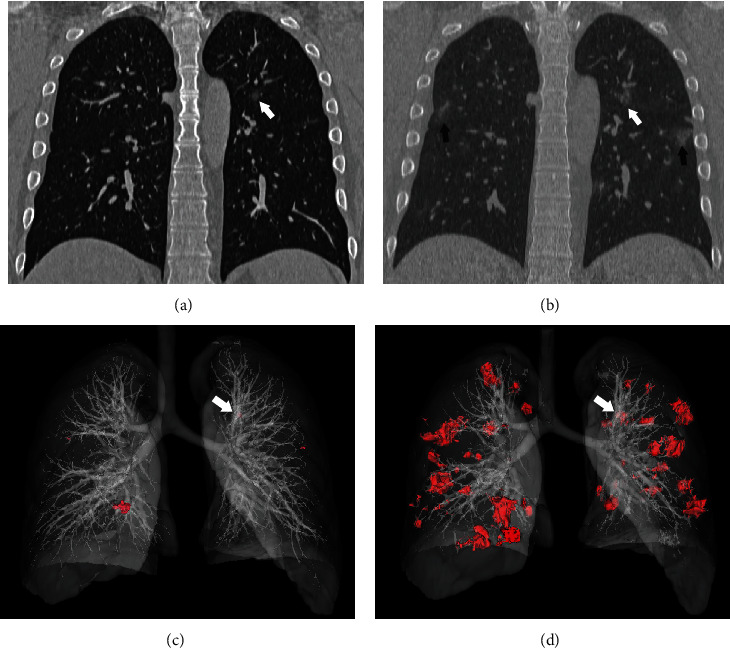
Imaging in a 25-year-old man who presented with fever and cough for 2 days. The coronal CT image shows a small ground-glass nodule (white arrow) in the apicoposterior segment of the left upper lobe (a). Three days later, the second CT image shows multiple nodular and patchy ground-glass opacities (black arrow) distributed bilaterally and peripherally. The volume of preexisting ground-glass opacities (white arrow) is increased (b). 3D reconstruction (c, d) shows the intrapulmonary changes during the time of the two CT scans.
